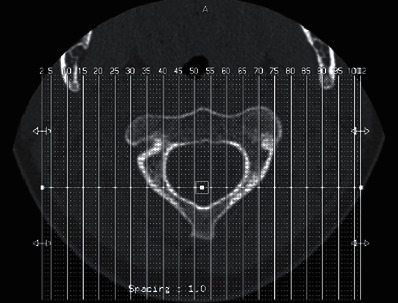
CT scans at follow-up of patient 1 show realignment of the atlas with the axis.
